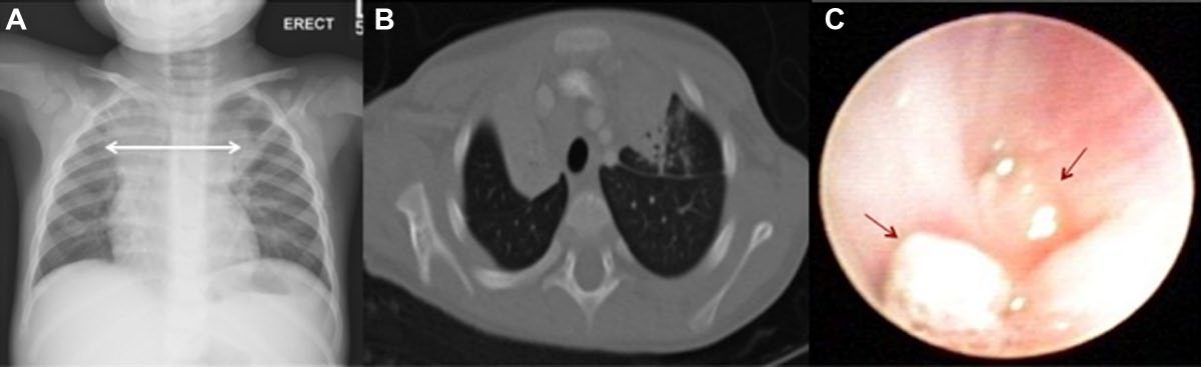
Radiographic and bronchoscopic findings. (A) Mediastinal widening on initial chest radiograph. (B) Extensive mediastinal lymphadenopathy, right main bronchus compression with right upper lobe collapse on chest computed tomography scan during hospital admission. (C) Endobronchial masses found during bronchoscopy. Mycobacterium *avium-intracellulare* complex was isolated from endobronchial granulation tissue.
